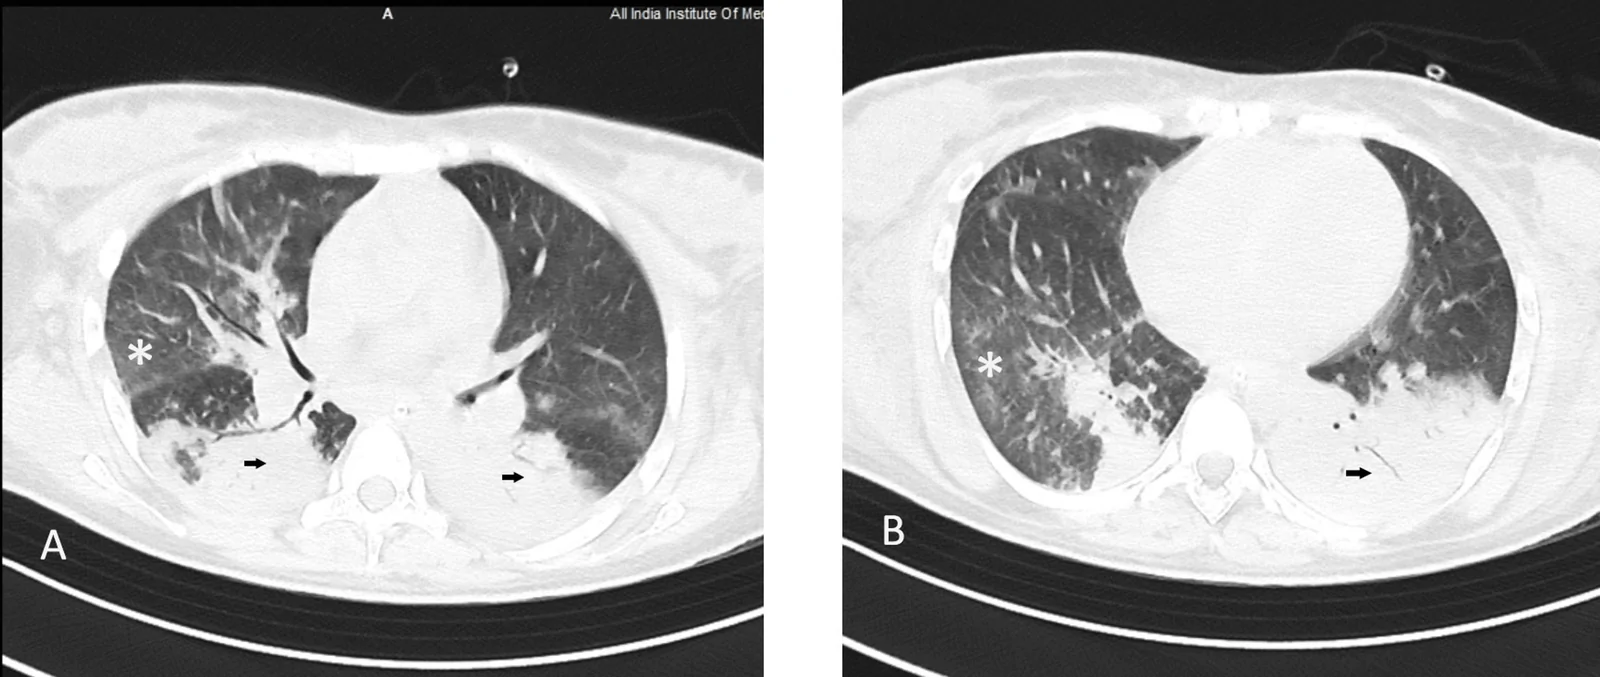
HRCT chest High-resolution computed tomography (HRCT) chest showing ground-glass opacities (asterisk) in the right upper and middle lobes, with areas of consolidation in the bilateral lower lobes (black arrows).
